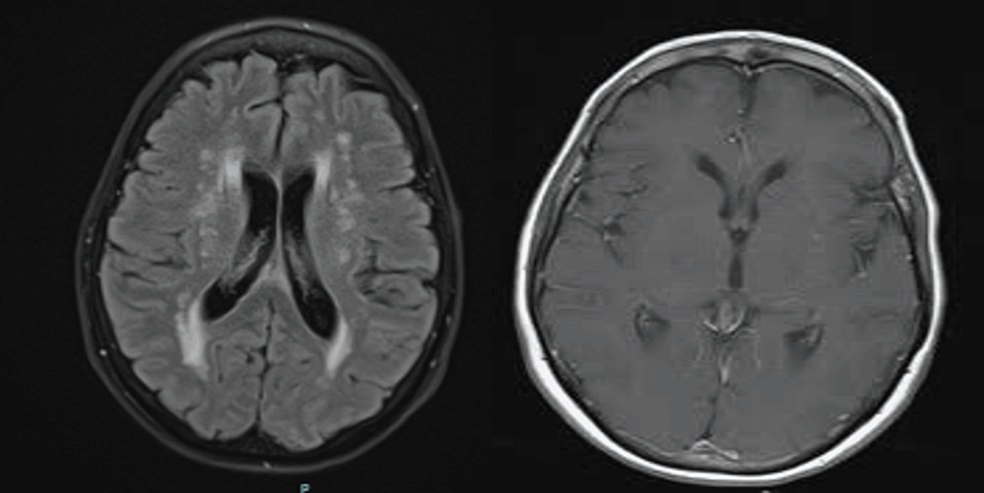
MRI brain wwo contrast. FLAIR (left) reveals periventricular white matter hyperintensities, and T1 with contrast (right) shows no contrast enhancement. wwo: With and without; FLAIR: Fluid-attenuated inversion recovery.
